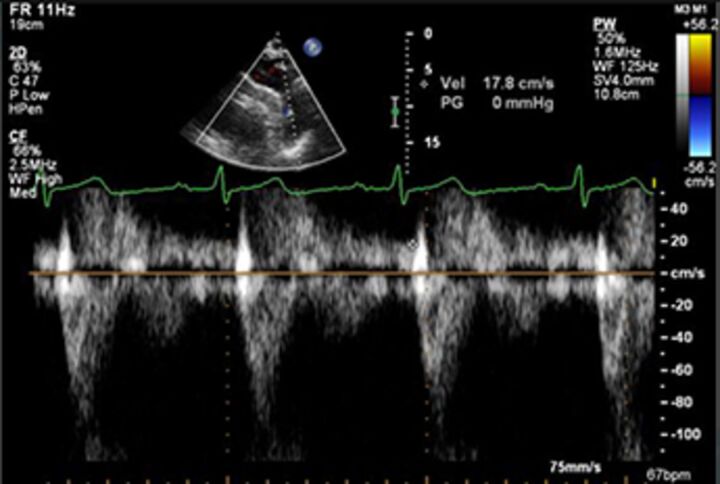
Easy If present in abdominal aorta, specific for severe AR	Less reliable when aortic stiffness increased (age) Depends on alignment of Doppler with jet direction Variable according to location of sample	Brief flow reversal is normal	Holodiastolic, end-diastolic flow Vmax>20 cm/s
